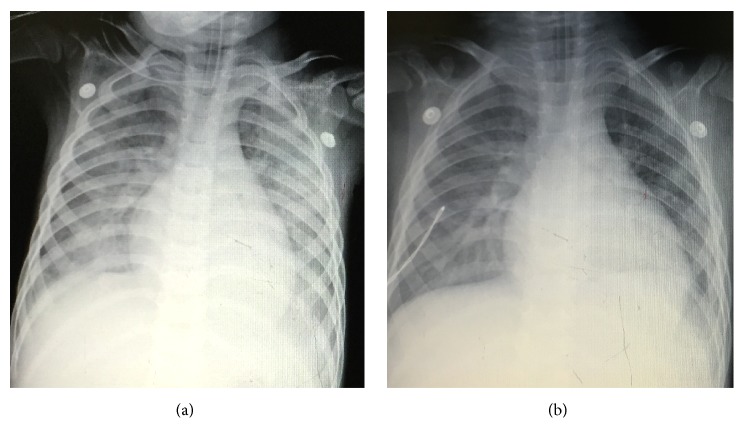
Chest X-ray exam on admission and the second day of case 1. (a) On admission, bilateral symmetrical infiltration response for pulmonary edema was revealed. (b) The second day, bilateral pulmonary edema was obviously decreased.
